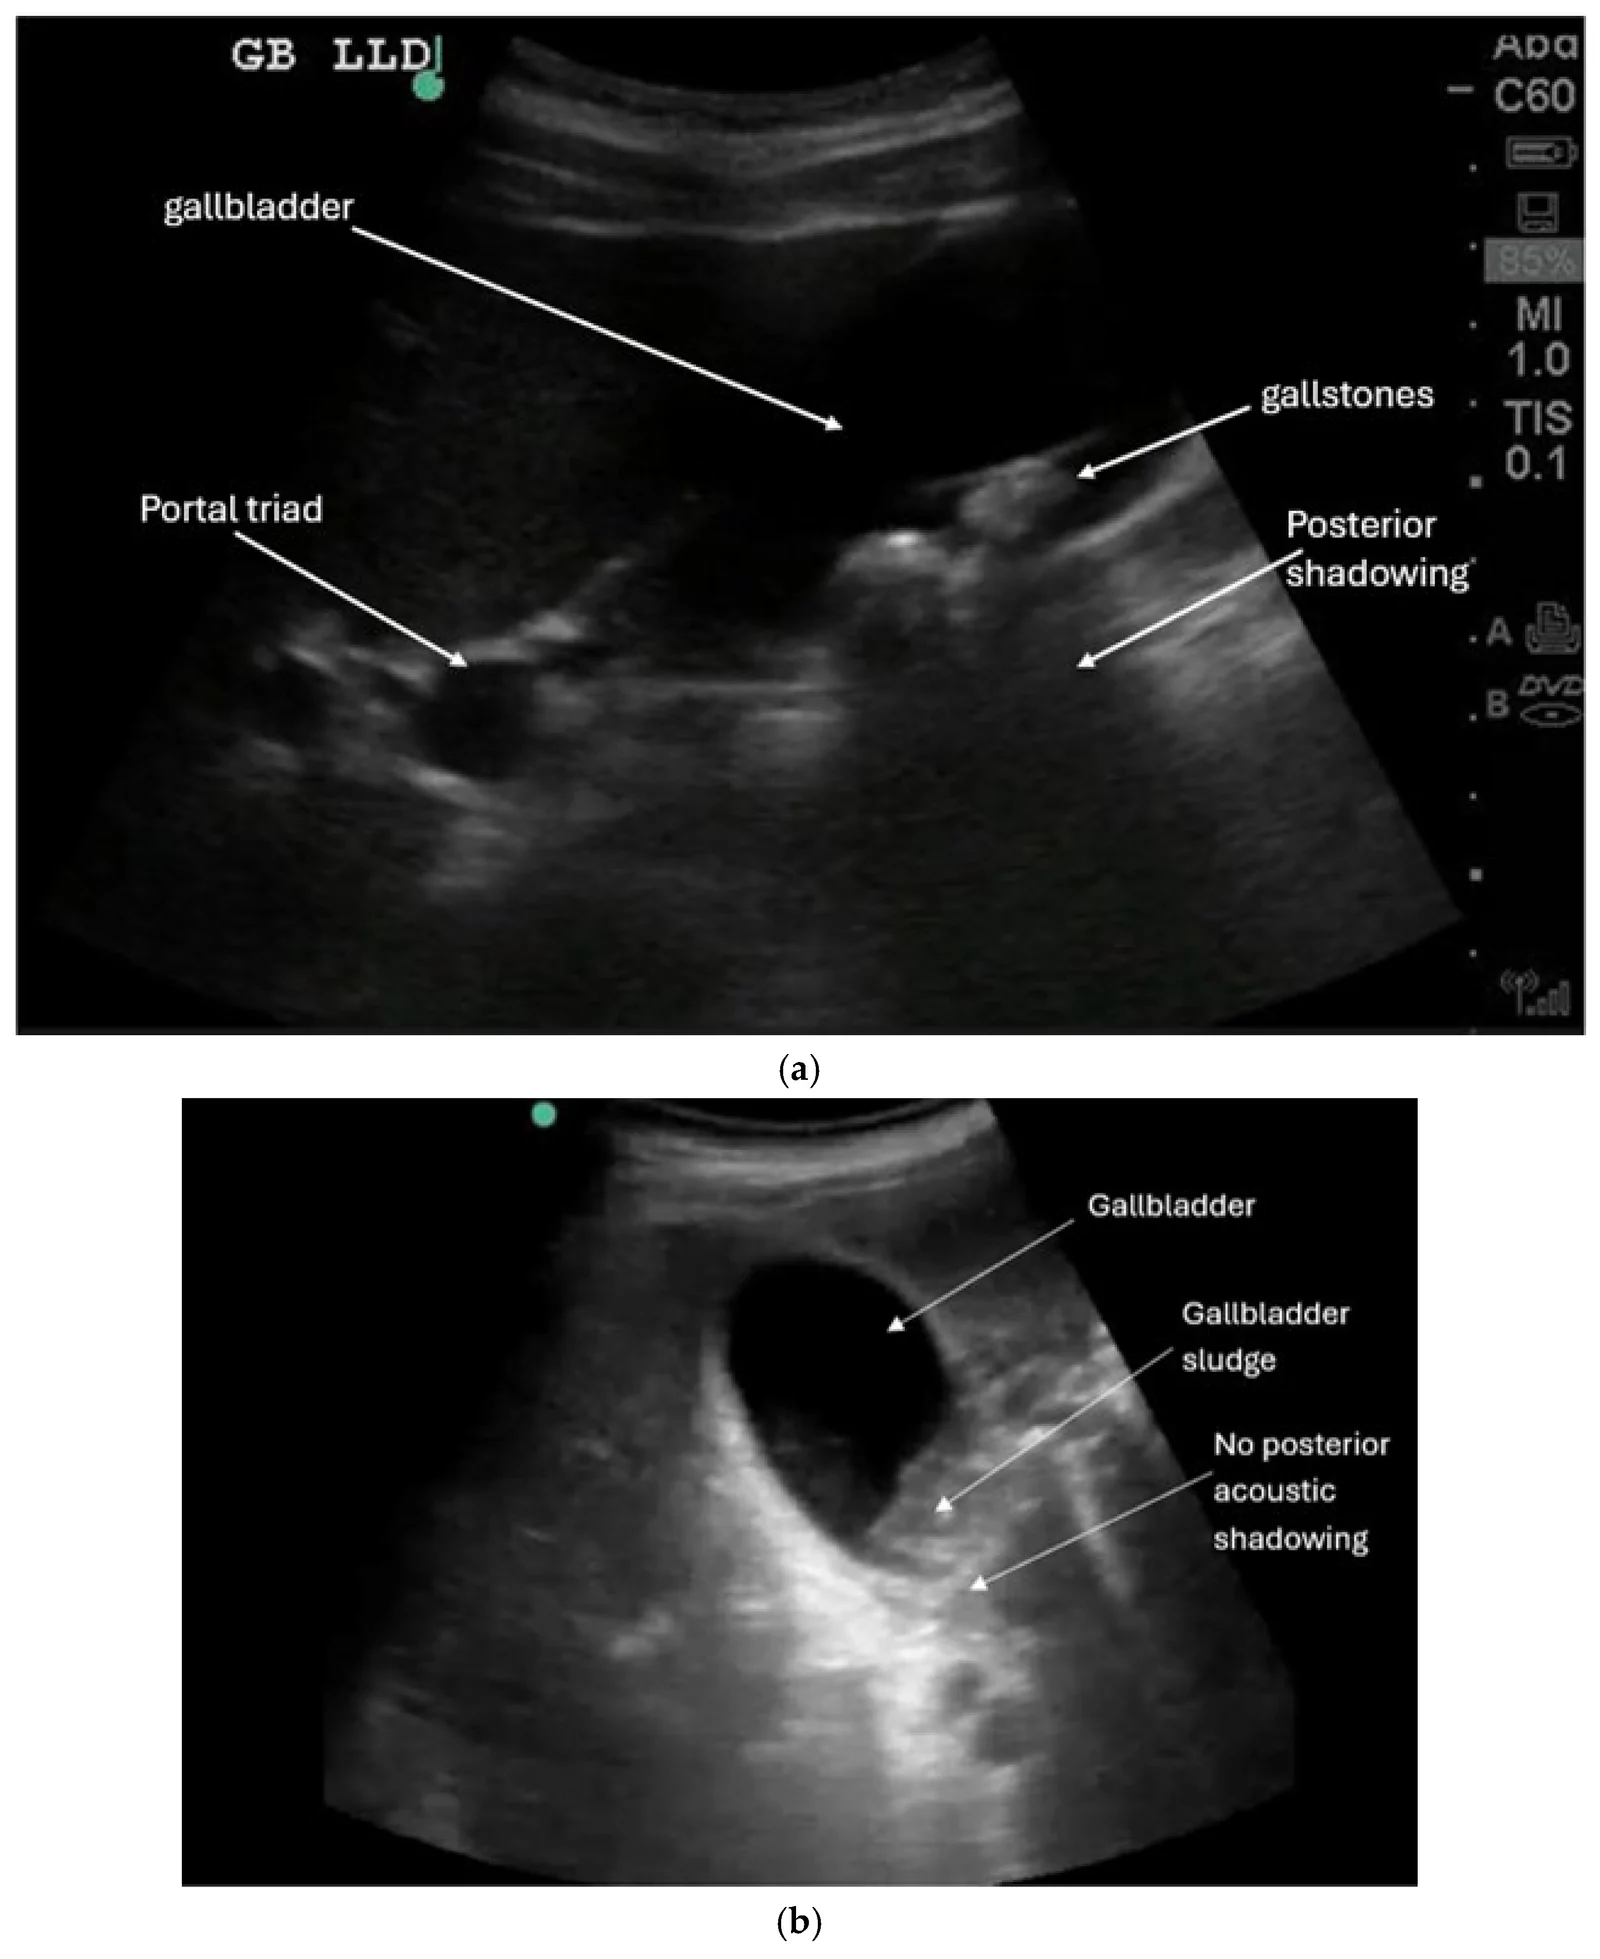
(a,b) Cholelithiasis is defined by the presence of gallstones within the gallbladder. It usually presents asymptomatically or with biliary colic pain when transient obstruction of the cystic duct occurs, often triggered by fatty meals [1,2,5,6]. POCUS has high sensitivity (96–97%) and specificity (97–99%) for detecting gallstones that are >1.5 mm diameter in size [5,6]. Using a curvilinear probe in the RUQ, the clinician should tilt the probe to view the entire gallbladder in a transverse plane. Then rotate the probe 90 degrees to view the gallbladder in a longitudinal axis. On ultrasound, gallstones usually appear as small or large round hyperechoic mobile structures with posterior shadowing due to their increased density (a). A video clip should be obtained in the LLD position to ensure gallstone mobility with patient position changes [3,4,5]. In (a), the portal triad is also visible, which contains the hepatic artery, portal vein, and the common bile duct. (b) is an example of gallbladder sludge, which can be present with or without gallstones. Sludge can be distinguished from stones because it does not have posterior acoustic shadowing or mobility. Patients with symptomatic cholelithiasis with severe pain and vomiting may warrant a surgical consult for admission. Otherwise, they can be evaluated outpatient with a general surgery referral for possible outpatient cholecystectomy.
